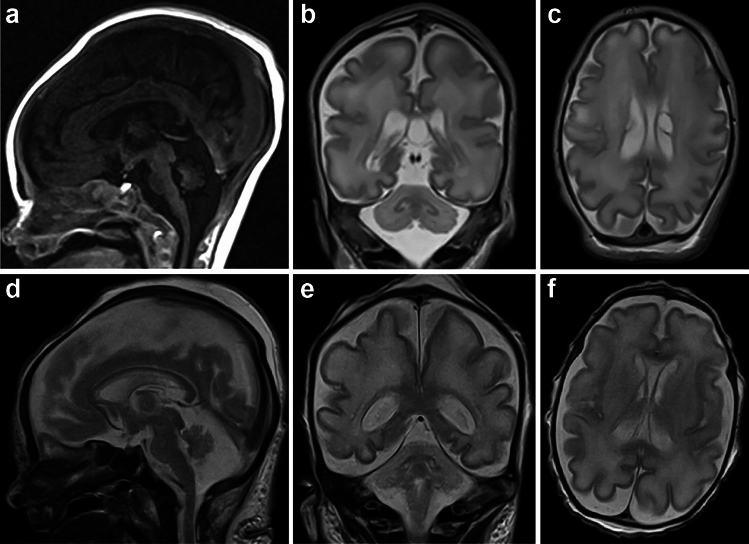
MRI of proband AII-1 and BII-1. a Midline sagittal FLAIR, b coronal T2 and c axial T2-weighted brain MRI of AII-1 at 7 days of age (corrected gestational age of 35/40 weeks), demonstrated a mildly small pons and pan-cerebellar hypoplasia. A cavum septum pellucidum and vergae, along with bilateral choroid plexus cysts, are visible in (c) (left: 9 × 6 mm; right: 6 × 3 mm; anteroposterior × transverse; mild gliotic lining of cysts observed during autopsy). d Sagittal, e coronal and f axial T2-weighted brain MRI of BII-1 at 12 days of age (corrected gestational age of 33 + 5/40 weeks) showing cerebellar vermian height, width and transcerebellar diameter are all more than 3 standard deviations below the mean for a 33-week-old corrected age neonate
